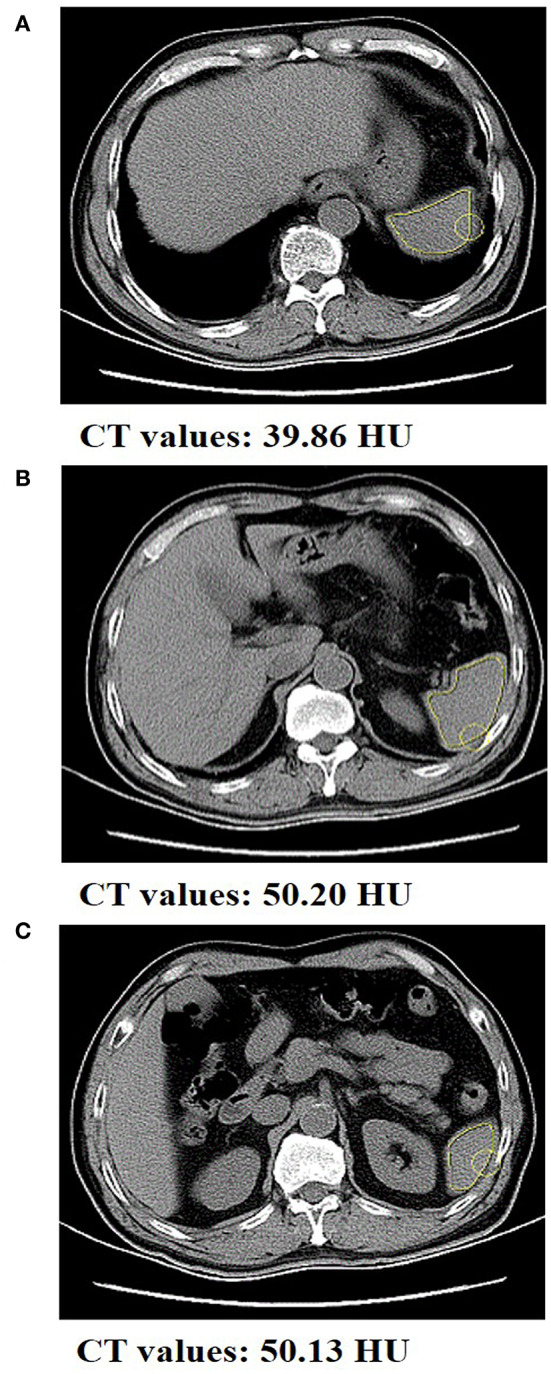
Abdominal CT scan of a gastric cancer patient with spleen density. The CT value of spleen at the (A) upper pole level, (B) the hilum level, and the (C) inferior pole level was 39.86, 50.20, and 50.13 HU, respectively.
